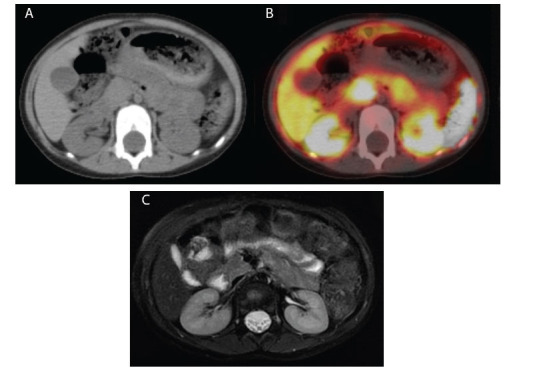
The CT scan (A) and matching Ga68-DOTATATE fusion image (B) of Case 3 demonstrate significant uptake on the pancreatic uncinate process. By using a diffusion-weighted MRI scan (C), which showed no abnormalities in the region, it was determined that the pancreatic region rich in SSR had physiologically high tracer uptake (SUVMax: 16.3).
